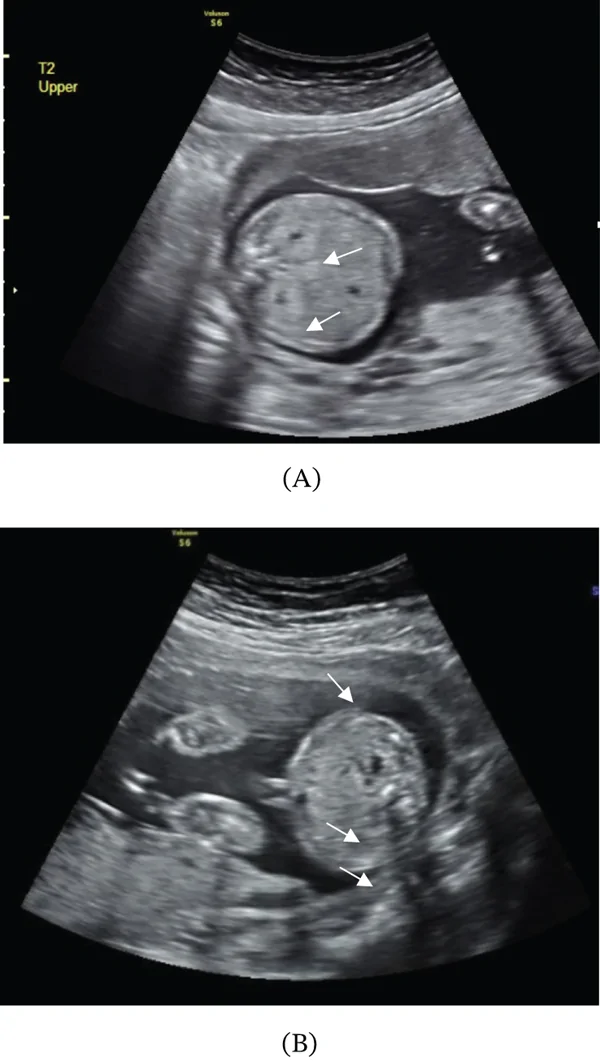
Ultrasound findings for (A) T2 and (B) T1. Both kidneys appearing brighter than cotwin suggesting polycystic kidney disease. Prenatal ultrasound findings at 20 weeks of gestation in a dichorionic diamniotic twin pregnancy. (A) Transverse ultrasound image of the affected twin (T2) demonstrating bilaterally enlarged, diffusely hyperechogenic kidneys (arrows), consistent with autosomal recessive polycystic kidney disease (ARPKD). Note the loss of corticomedullary differentiation and the relatively reduced amniotic fluid volume compared with the cotwin. (B) Transverse ultrasound image of the unaffected cotwin (T1) demonstrating normal renal echogenicity and architecture (arrows) with normal amniotic fluid volume, included for comparison.
